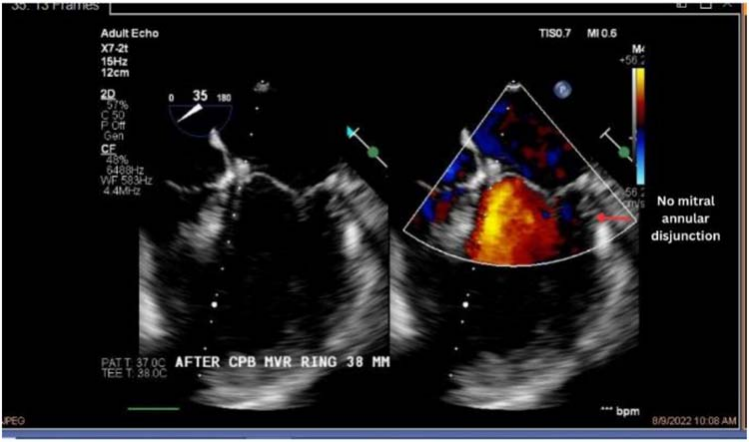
Postoperative echocardiography of patient 1.
